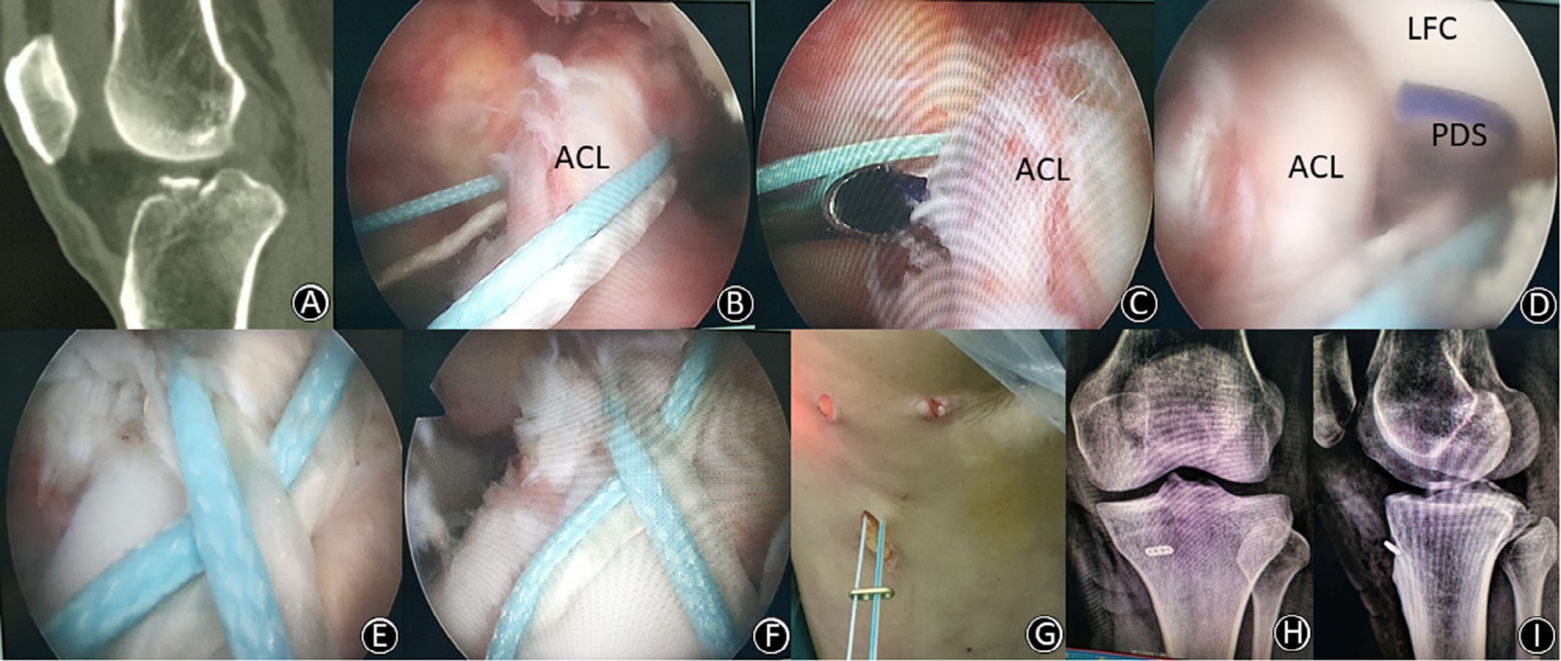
(A) A 45‐year‐old woman suffered a displaced anterior cruciate ligament (ACL) avulsion fracture (sagittal CT image) of her left knee caused by a road‐traffic accident. (B) UltraBraid sutures were passed from the medial side of the ACL, around its back, to its posterolateral side. (C, D) A guide PDS was placed *via* a 45° SutureLasso, through the fibers of the ACL, close to its tibial bony insertion, posterior to its mid‐coronal plane, from medial to lateral. (E, F, G) UltraBraid sutures were passed through two bone tunnels (being drilled in) and tied over a button on the outer cortex of the tibia; then the figure‐of‐eight fixation was completed. (H, I) Postoperative anteroposterior and lateral plain radiographs showed anatomic reduction of the fragment.
